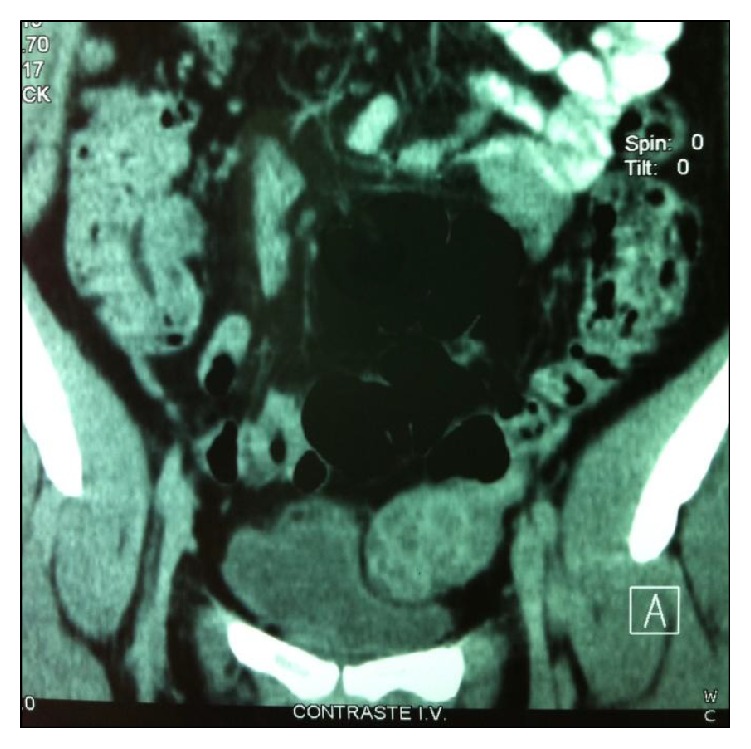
Contrast-enhanced abdominal CT revealing tumour on left ovary.
